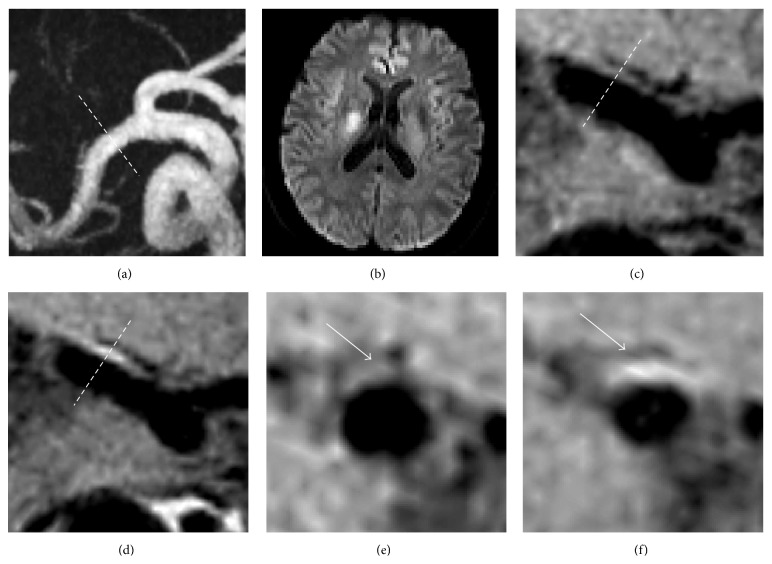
A 59-year-old man with left-side weakness. (a) MRA shows no stenosis on right MCA; (b) the diffusion-weighted image shows a hypertensive lesion in the right lenticular nucleus which extends to the coronal radiate; precontrast T1-weighted SPACE images did not show eccentric wall thickening clearly on both the long axis (c) and short axis ((e), arrow) of the MCA; postcontrast T1-weighted SPACE images show wall thickening ipsilateral to the infarct on both the long axis (d) and short axis ((f), arrow) of the MCA.
